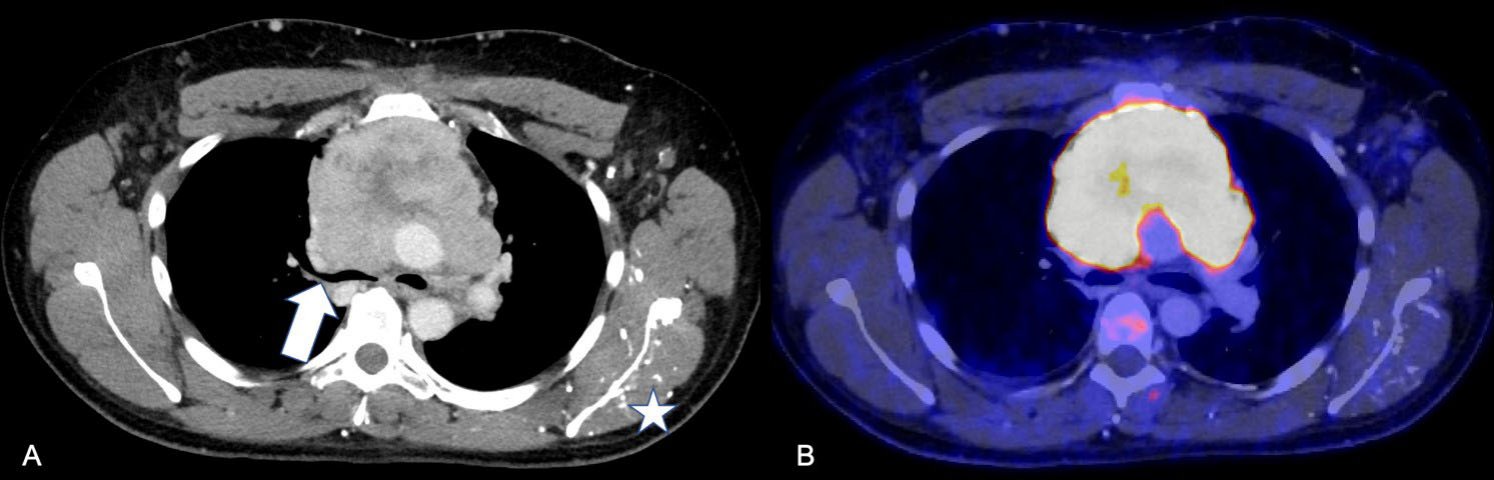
Radiological findings upon initial diagnosis of thymic carcinoma (A, B) Baseline hybrid PET/CT: (A) Axial contrast-enhanced CT scan demonstrates the large thymic carcinoma mass with sub-total collapse of main bronchi and superior vena cava syndrome (arrow). Influx of i.v. contrast agent applied in left cubital vein via muscular and thoracical veins (star). (B) Corresponding 18F FDG-PET fusion overlay indicates high metabolic activity of the tumor (yellow).
